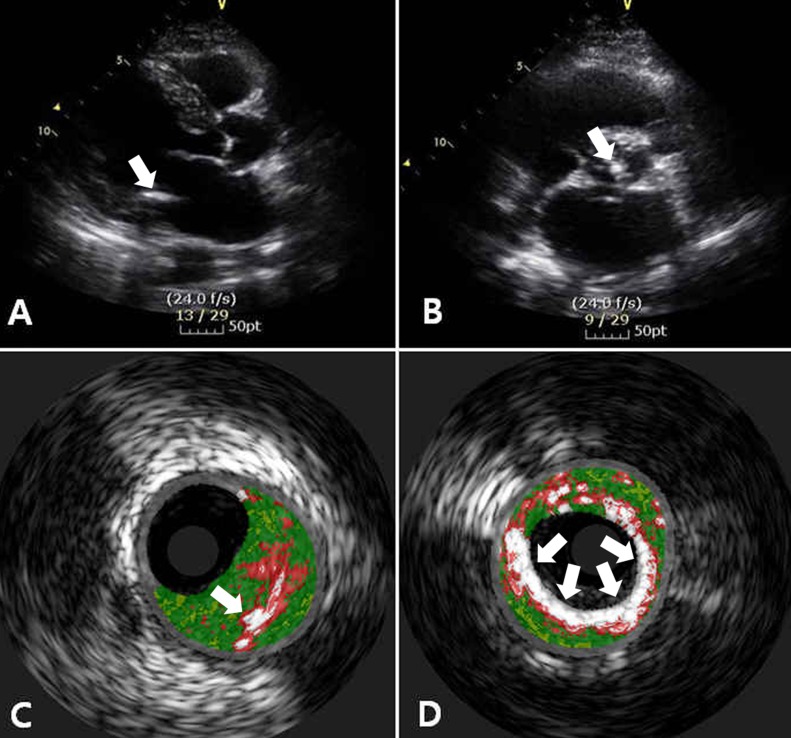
Representative images of valvular calcification and coronary artery calcification(white arrows). Transthoracic echocardiography showed mitral annular calcification (A) and aortic valve calcification (B). VH-IVUS demonstrated mild and severe coronary calcification. Mild valvular calcification revealed the prominent fibrous (green color) plaque components (C), while severe valvular calcification showed densely calcified plaque (D).
